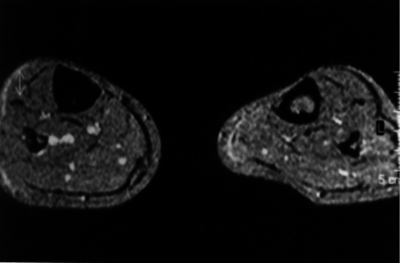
A 5-mm-thick axial T1-weighted image through the mid-tibia confirming massive endosteal sclerosis that caused obliteration of the canal of the right tibia.
